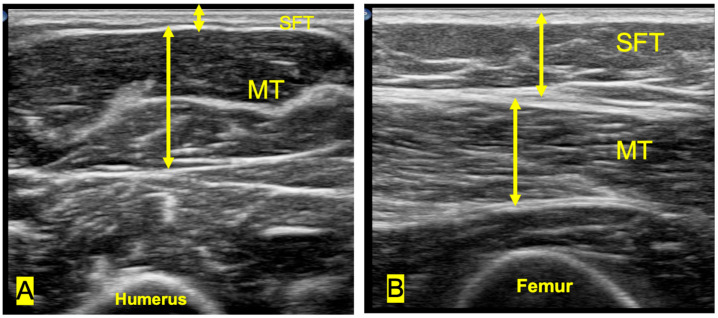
(A) Transverse ultrasonographic image of the biceps brachii muscle. (B) Transverse ultrasonographic image of the rectus femoris muscle. MT, muscle thickness; SFT, subcutaneous fat thickness.
